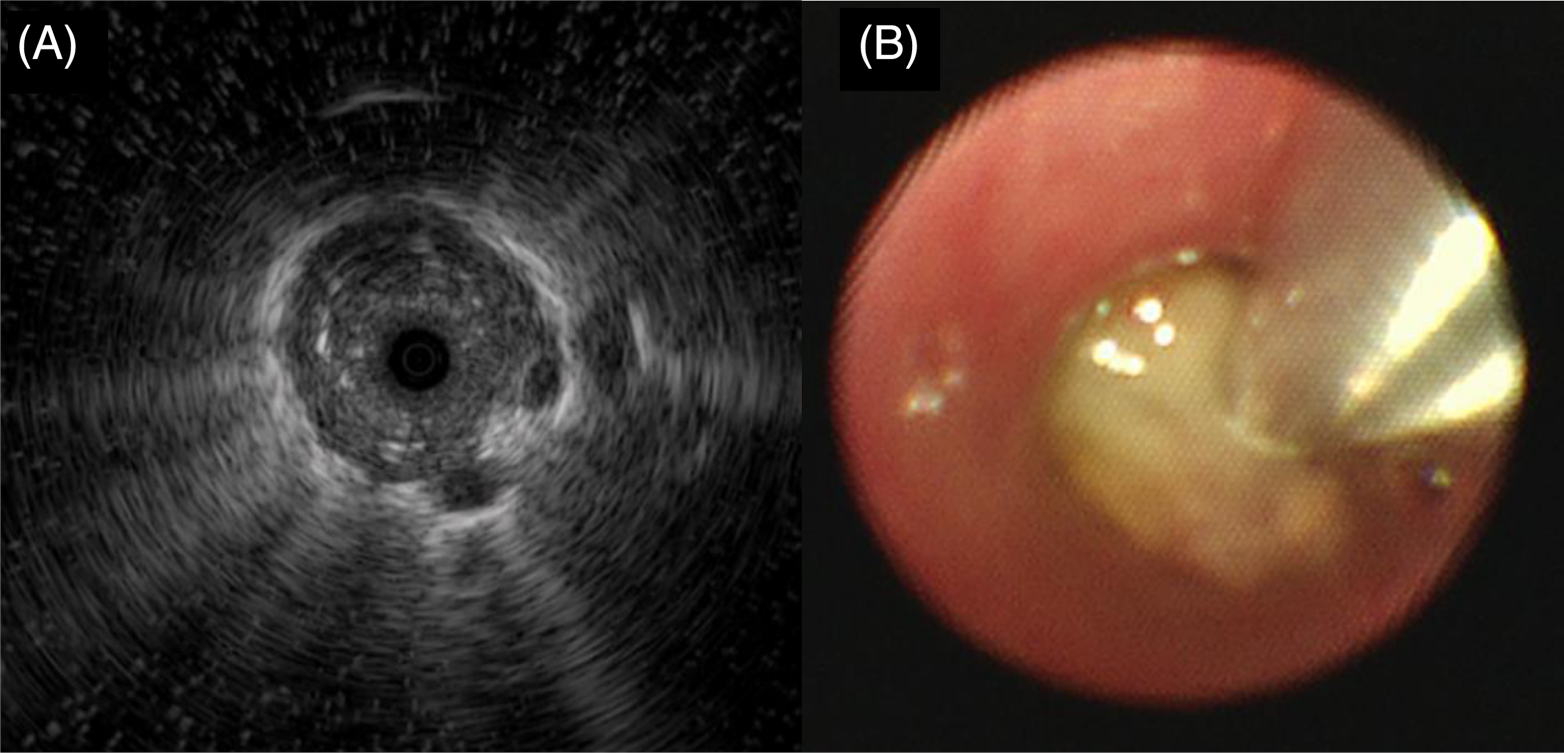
An ultrathin bronchoscope was advanced into the left B^5^ bronchi. A radial endobronchial ultrasound probe confirmed within the lesion (A). The bronchoscopy image in a well‐inhaled state revealed a yellowish tumour, indicating a mucus plug (B). These findings led to the diagnosis of allergic bronchopulmonary mycosis.
